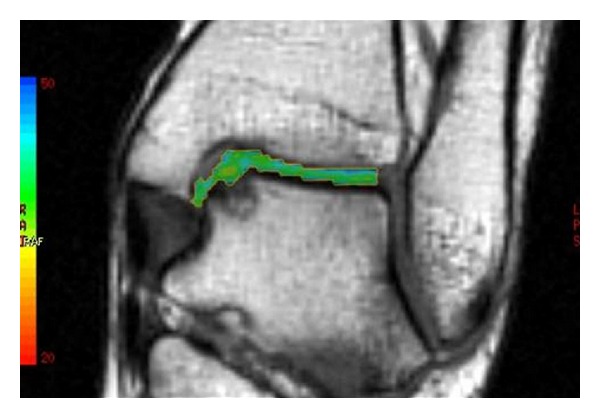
The T2 mapping MRI performed one year after the second treatment shows a 42 msec value, a sign of hyaline cartilage regeneration.
